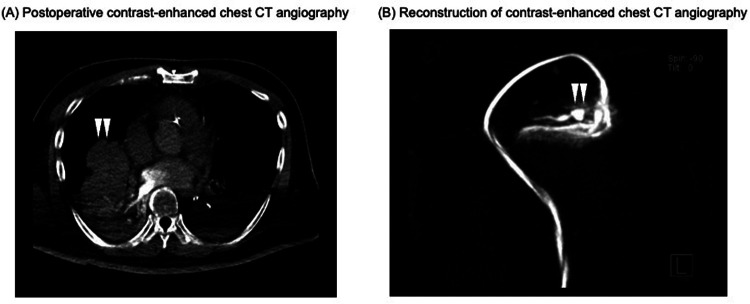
Postoperative contrast-enhanced chest CT angiography on POD 7. (A) Postoperative contrast-enhanced chest CT angiogram. The white arrows indicate atelectasis surrounding a pseudoaneurysm in the right middle lobe branch. (B) Reconstruction of contrast-enhanced chest CT angiogram at the site of the pseudoaneurysm and surrounding vessels. The white arrows indicate the site of the pseudoaneurysm. CT, computed tomography; POD, postoperative day.
